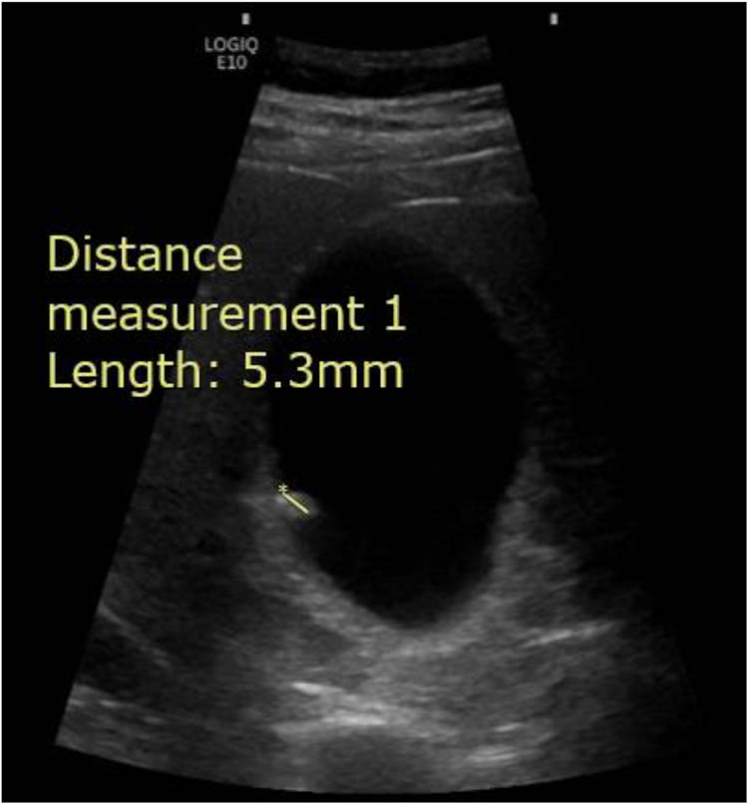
Abdominal ultrasound demonstrating the presence of a 5.3 mm stone within the gallbladder.
